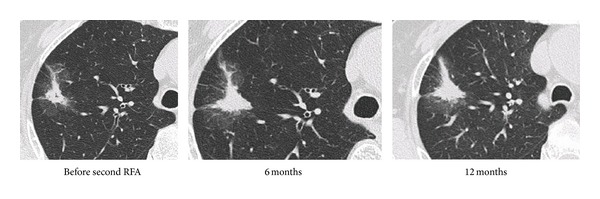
Serial CT findings before and after second bronchoscopy-guided cooled radiofrequency ablation (RFA) in case 1.
